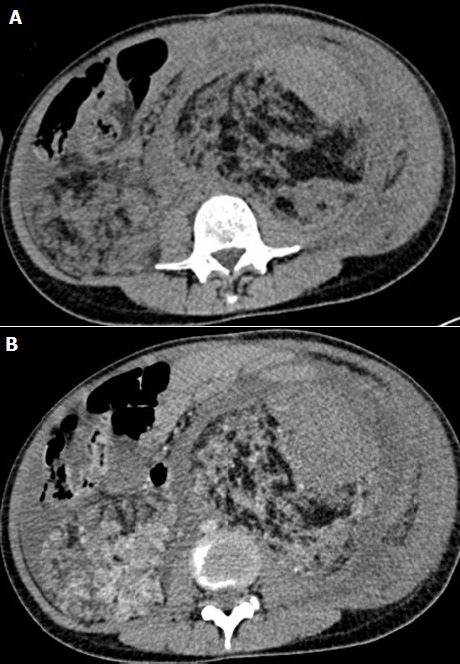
(A,B) axial abdominal computed tomographic scan showing bilateral abdominal masses of fat attenuation that replace and displace renal parenchyma bilaterally, with a lesion of soft tissue attenuation and whorled configuration of hyper attenuating fluid that suggests recent intra-tumoral hemorrhage in the left kidney
